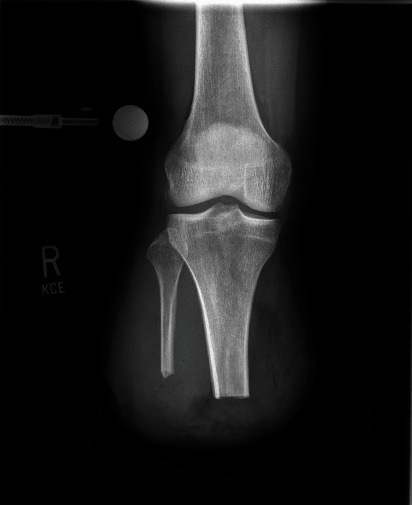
Anteroposterior radiograph of the right knee fourteen days postoperatively showing transtibial BKA.
